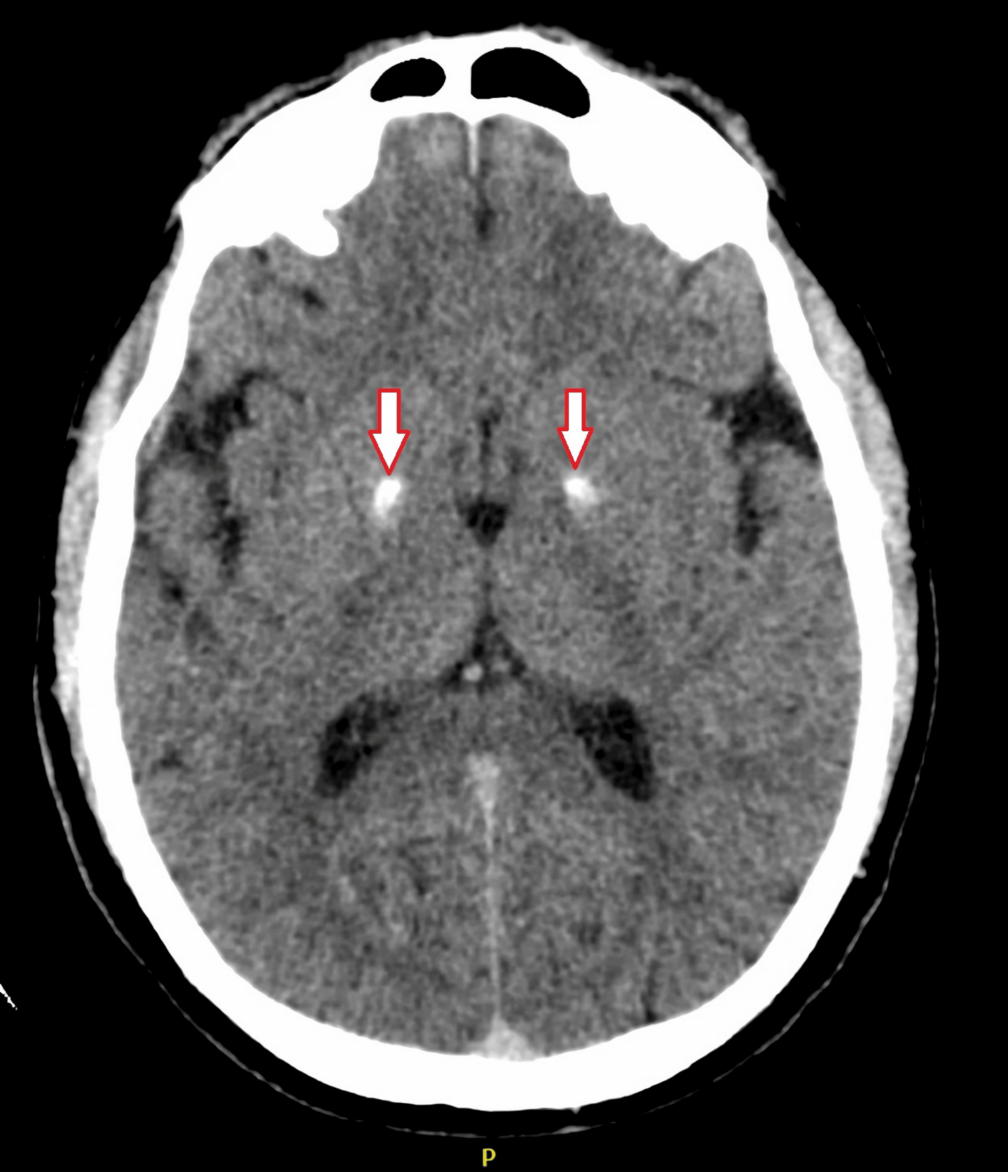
Non-contrast axial computed tomography (CT) of the brain showing bilateral symmetrical hyperdensities in the globus pallidus (arrows), consistent with calcium deposition.
